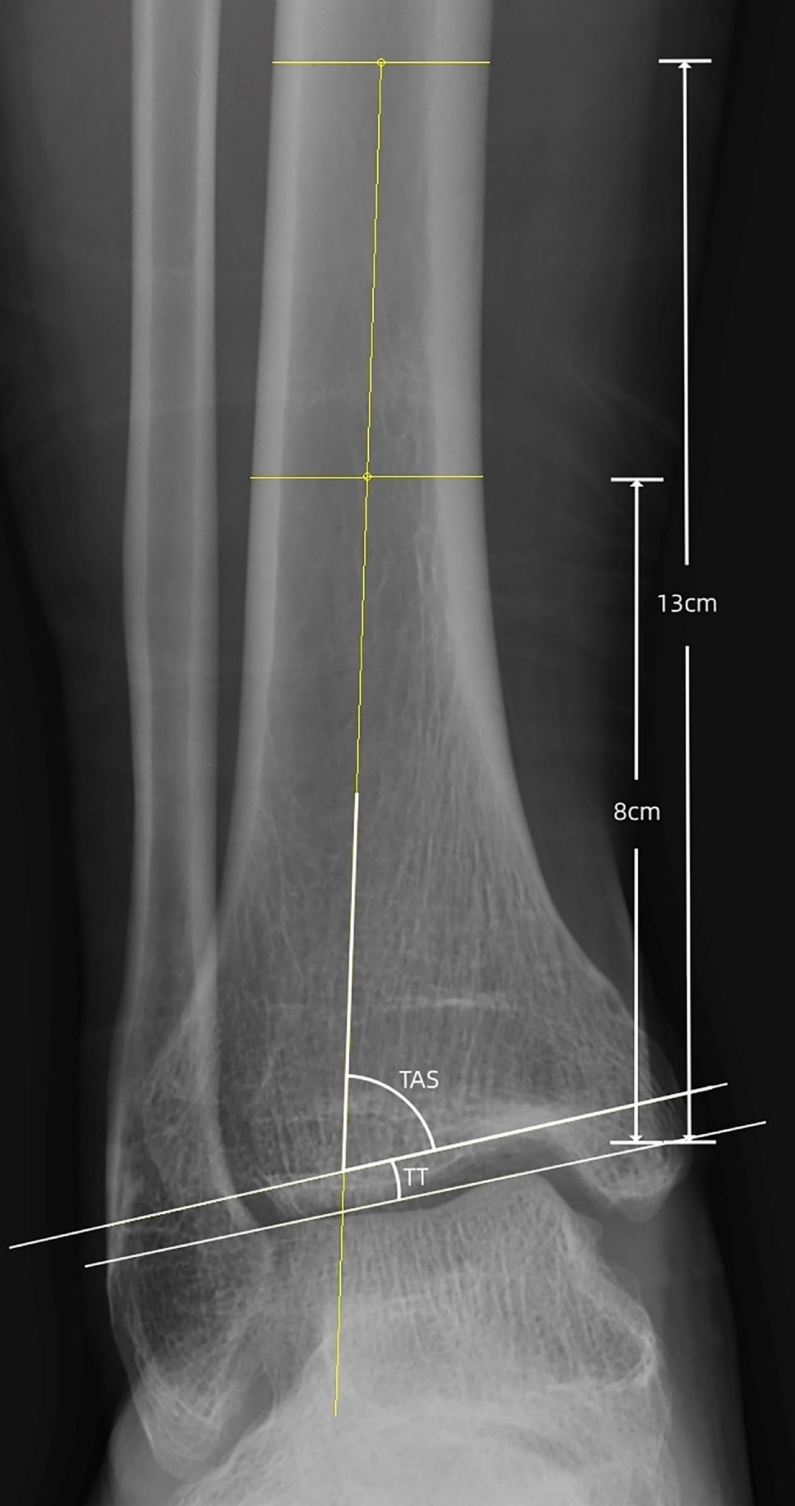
Radiographic parameters. The tibial axis was characterized by a line connecting the midpoints of the tibial shaft at 8 cm and 13 cm proximal to the medial malleolus tip. The angle formed between the distal tibial articular surface and this tibial axis defines the Tibiotalar Surface Angle (TAS). The angle between the superior talar articular surface line and the distal tibial articular surface constitutes the Talar Tilt (TT) angle
